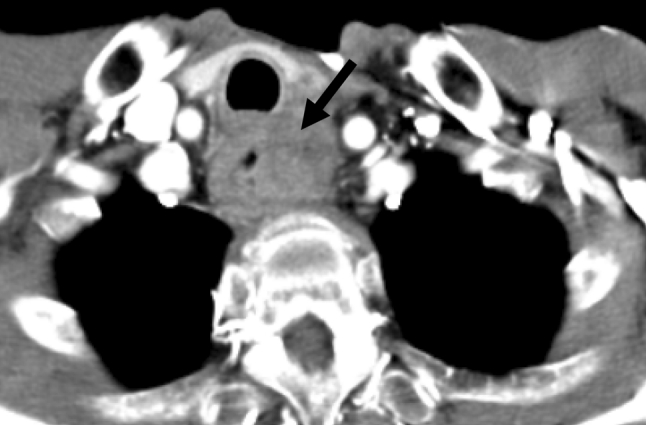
Contrast-enhanced axial CT image a week before the stent placement shows the esophageal cancer (*arrow*) in the cervical region in a 64 year-old man
